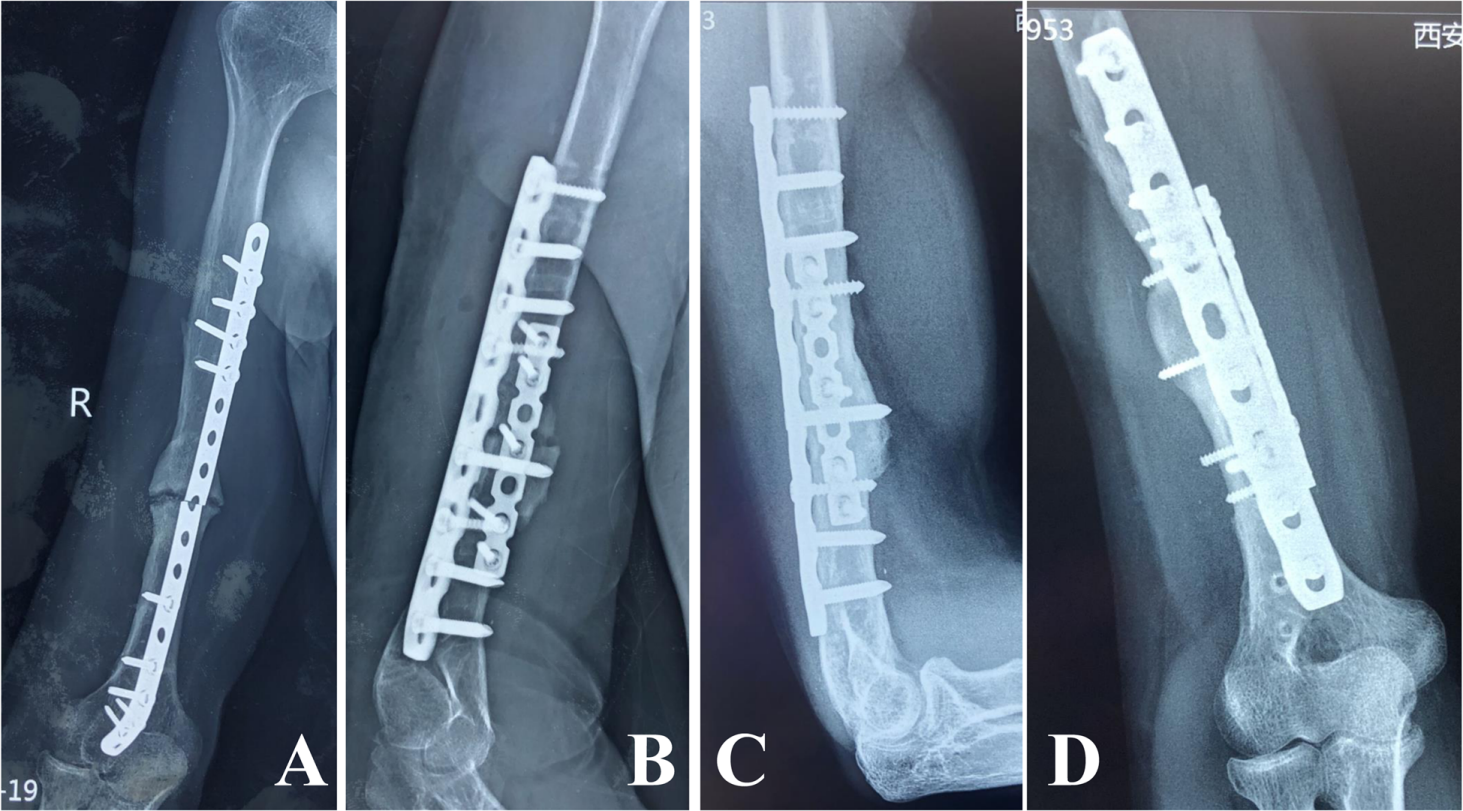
Images from a 42-year-old man with right humeral shaft nonunion for more than 26 years who had undergone four surgeries before seeking treatment at our institution. a Radiograph showing nonunion with implant failure at 4 years after the last revision. b Fixation using double locking compression plating and bone grafting. c, d Radiographs demonstrating osseous union at 8 months postoperatively
